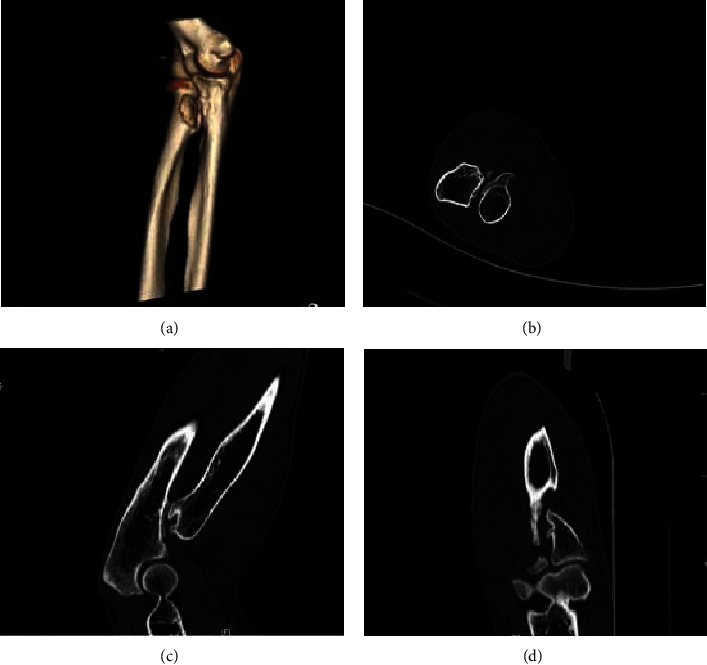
Based on the 3D image reconstruction, the right radial bone is locally irregularly raised, the internal density is unevenly reduced, the CT lesion is continuous with the surrounding cortex, and the interior is cartilage density or signal.
